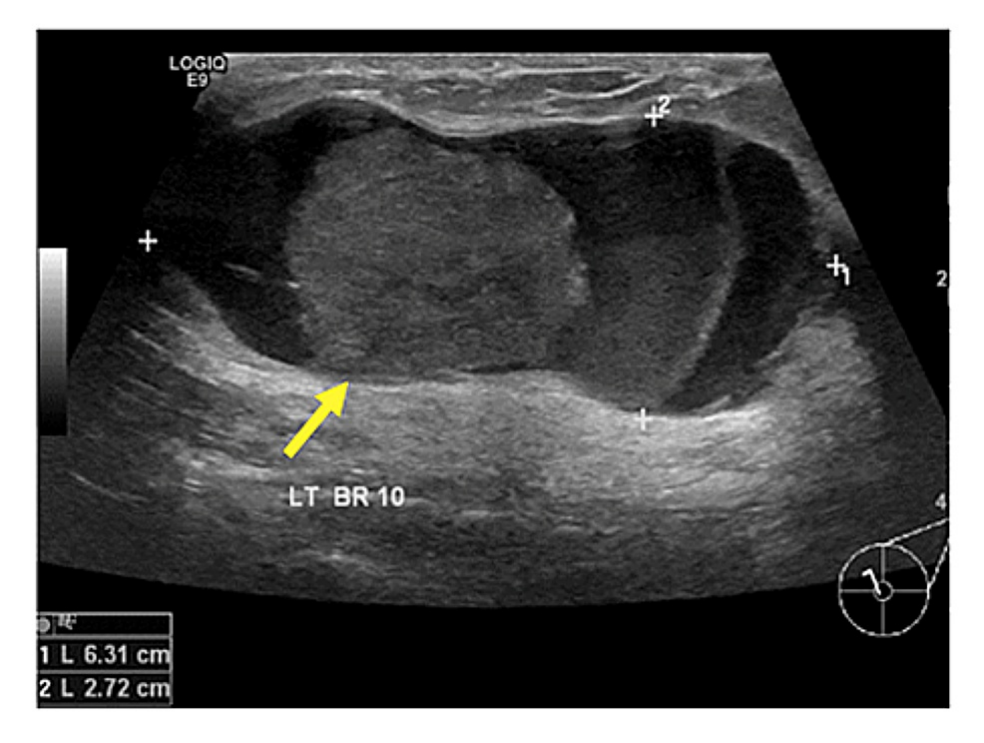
Pathology-proven papilloma with ultrasound finding of a solid lesion within a dilated duct (yellow arrow).
